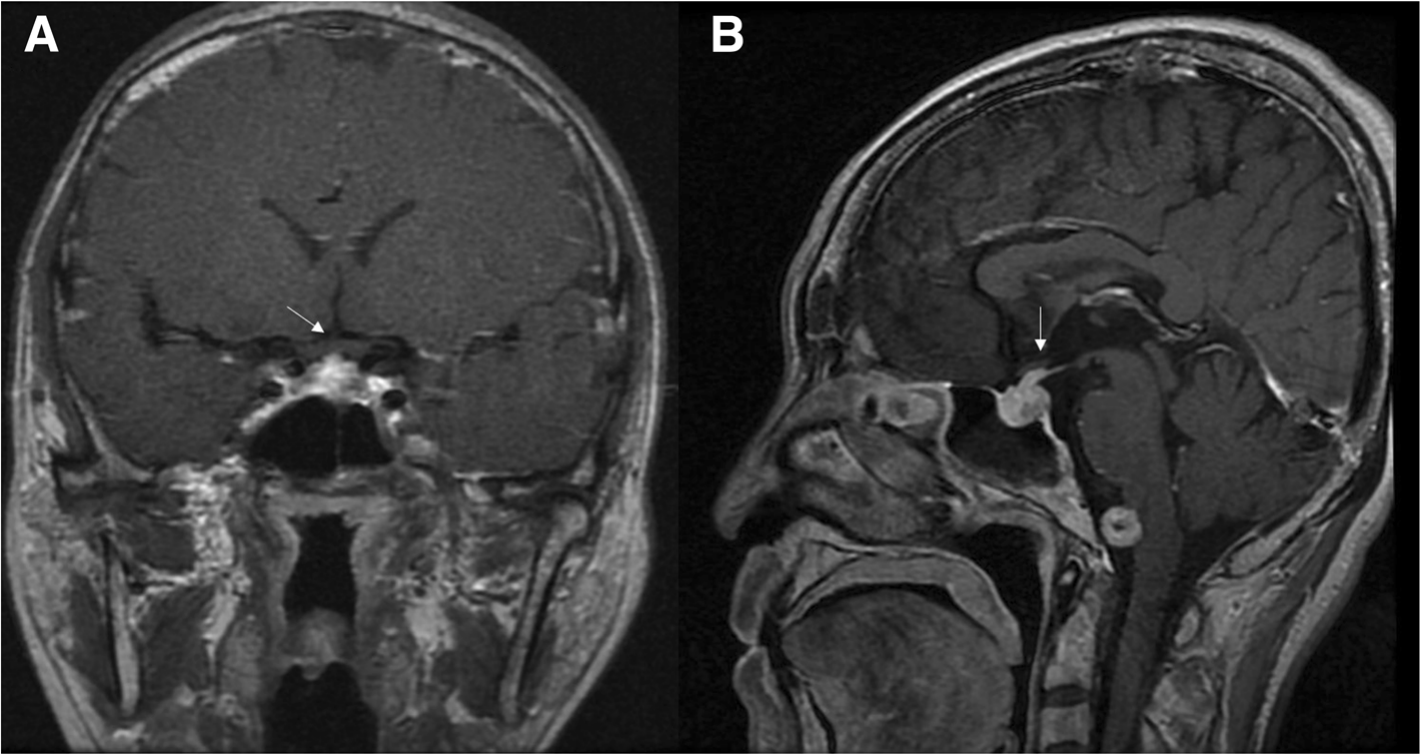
IgG4-RD with hypophysis involving optic chiasma. coronal (a) and Sagittal (b) contrast enhanced T1-weighted MRI showed diffuse enlargement of the pituitary and infundibulum, resulting in the compression of optic chiasm. White arrows show the opposition of optic chiasm
